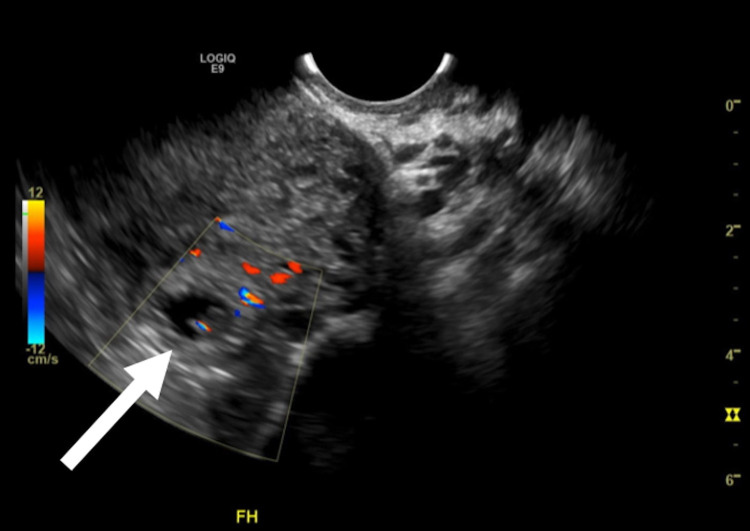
Six-week pregnancy on ultrasonography showing pregnancy (arrow) within the left fallopian tube stump.
